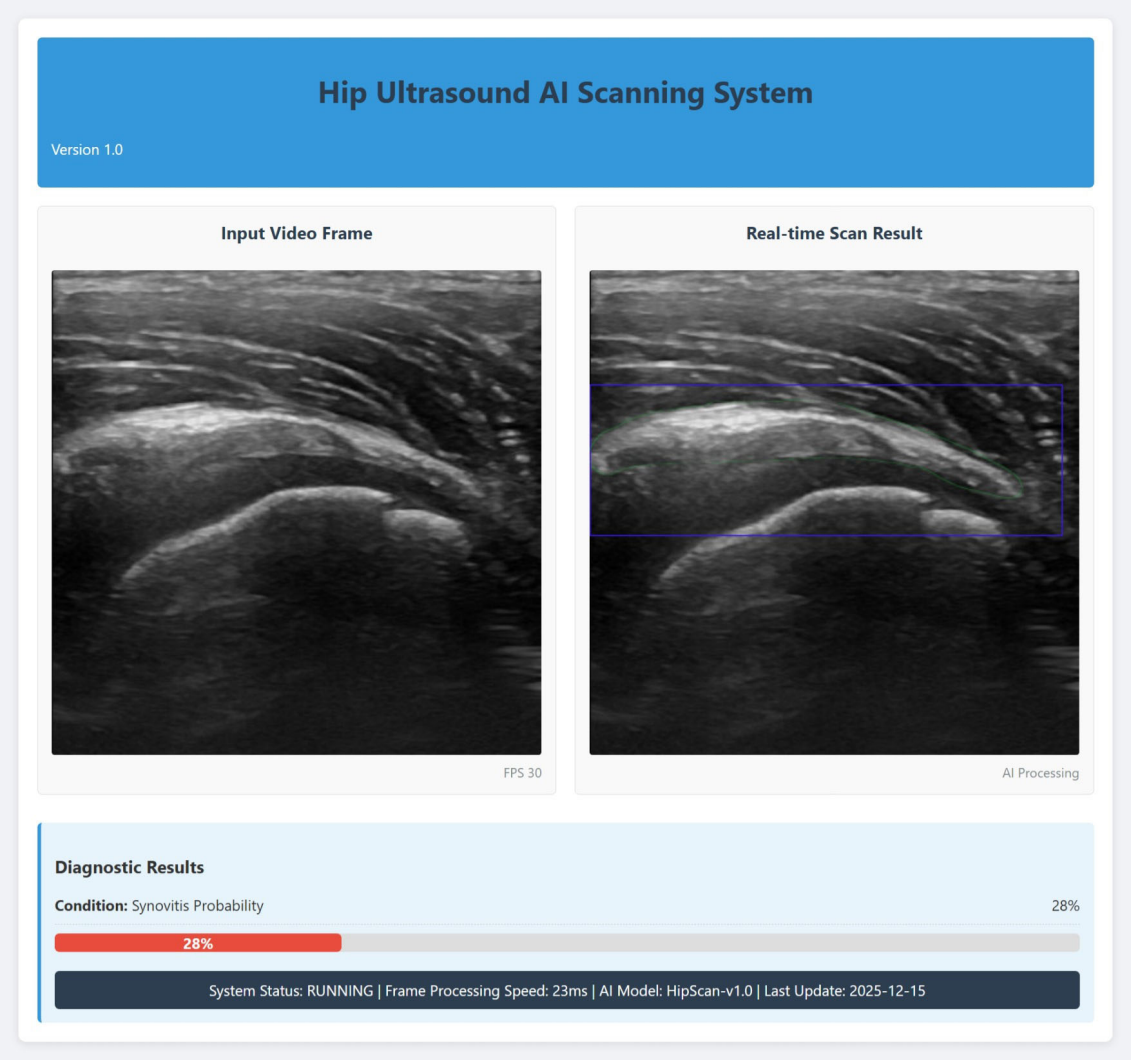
Hip ultrasound AI scanning system: Real-time frame-by-frame analysis and synovitis risk assessment.
